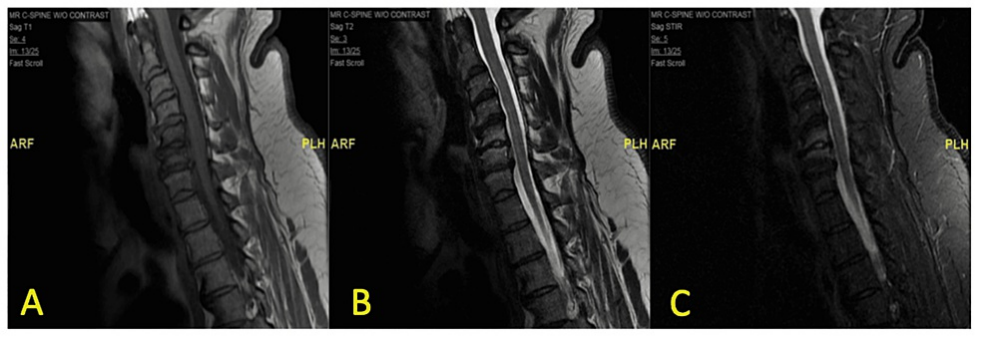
MRI of the cervical spine Sagittal view of the cervical spine demonstrates hypointense T1 (A) and hyperintense T2 (B) signals in the vertebral bodies of C5 and C6 as well as short tau inversion recovery (STIR) signal/postcontrast enhancement (C) in the inferior half of the C5 vertebral body and the superior half of the C6 vertebral body, consistent with osteomyelitis and discitis.
